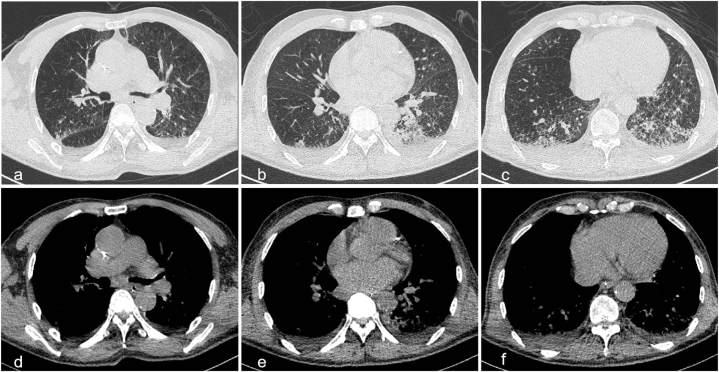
Repeat chest CT scan on day 2 after ICU admission. (a–c) The lung window images. (d–f) The mediastinal window images. CT, computed tomography; ICU, intensive care unit.
